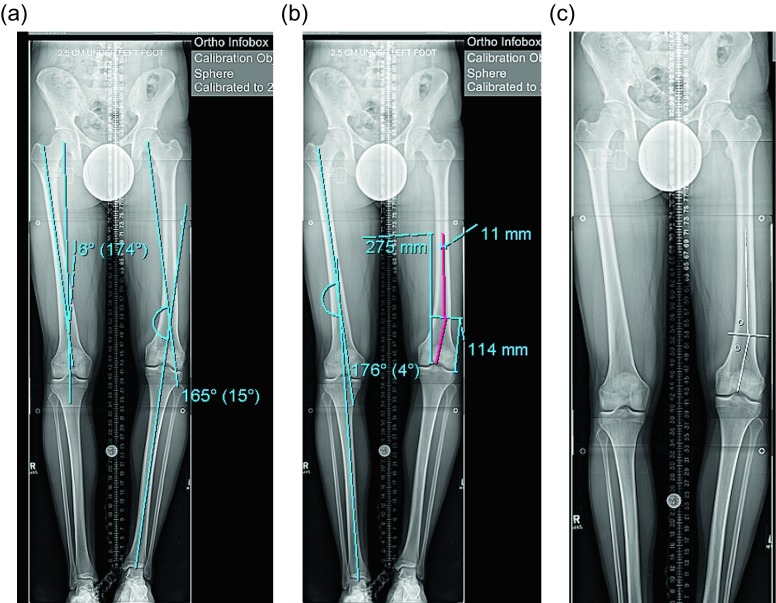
(a) This 51 inch AP radiograph allows for deformity planning with correction of varus and shortening. The mechanical axis planning is used to determine the magnitude and location of the deformity. (b) The red lines show the planned path of the ILN that will ensure correction of mechanical axis. The lines also show the length of the nail. (c) The white lines are placed to designate the path of the nail, and the circles are used to mark the location of the blocking screws. These radiographic plans are brought into the OR for comparison with intraop fluoroscopy shots.Osteotomy site: The osteotomy site that was selected on the preoperative plan is located on the femur under fluoroscopy. A 10 mm incision is made in the lateral thigh at this level and taken through the iliotibial (IT) band. The incision can be extended for an IT band release. We release the IT band routinely for correction of valgus and lengthening and for large lengthenings (over 4 cm). A drill is then inserted with a protective sleeve, and multiple drill holes are created in one plane. This creates vent holes during reaming and marks the osteotomy site to help with blocking screw placement.Blocking screws: When correcting coronal plane deformity blocking screws are inserted. The position of the screw needs to be decided as a part of the preoperative planning and replicated in the OR. For valgus deformity a blocking screw is placed lateral to the proposed path of the ILN (Figure 3a). For varus deformity the blocking screw is placed medial to the path of the nail (Figure 3b). An additional screw can be placed in the proximal fragment as well for optimal control. A blocking screw needs to be placed posterior to the path of the ILN (Figures 3c and 3d).Figure 3. F3:
